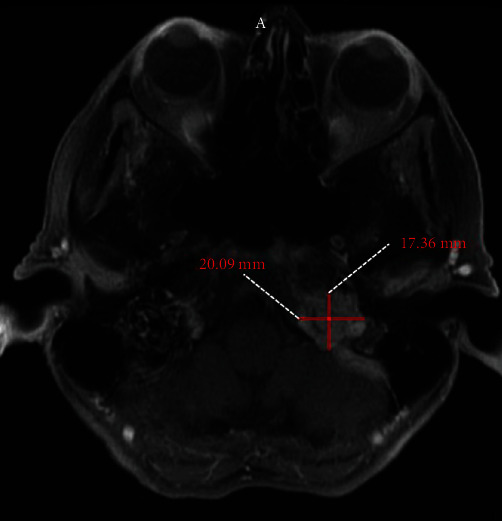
MRI of the neck with and without contrast showing a mass lesion centered within the left jugular foramen, appearing mildly increased in size. The size was 20 mm in maximal dimension as opposed to 17 mm compared to previous imaging 6 months prior.
